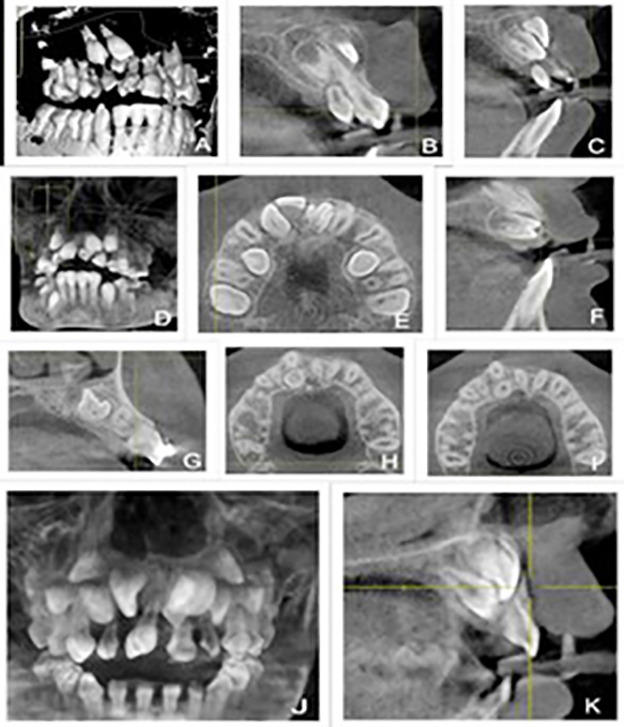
CBCT Representations of Tuberculate Supernumerary Teeth Showing Pulp Horn Variations and Root Development Stages.
